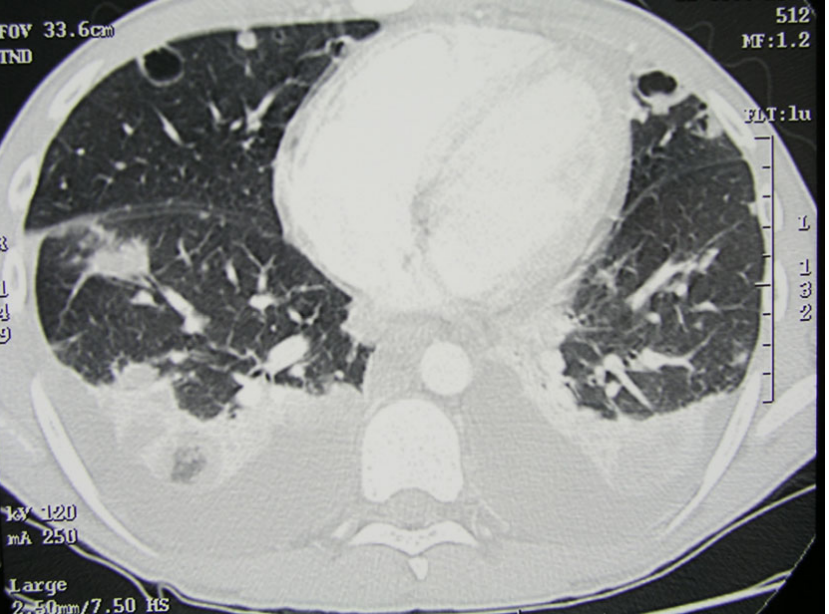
Chest CT of a patient with necrotizing pneumonia caused by CA-MRSA USA300 genotype. The CT scan, obtained on hospital day 5, shows multiple nodular lesions, some with a central cavitation, and bilateral pleural effusion.
